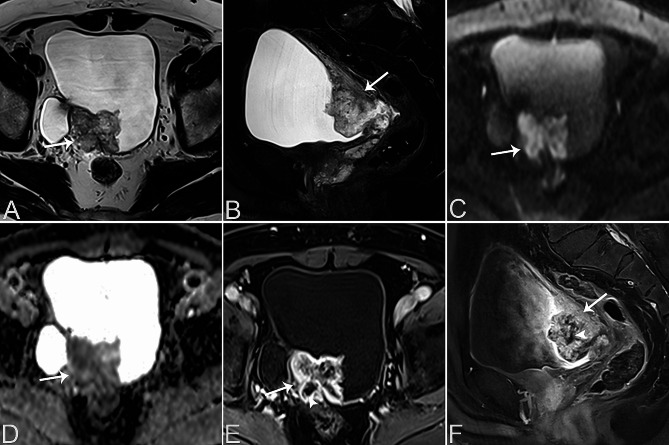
A 70-year-old male patient with bladder SUC in the right posterior wall of the bladder. Axial T2WI (A) and sagittal T2WI (B) show a lobulated mass in the right posterior wall of the bladder, demonstrating hyperintensity on T2WI (arrows). Axial DWI (C) shows the mass with high signal intensity (arrow). Axial ADC map (D) shows diffusion restriction of the bladder tumour (arrow). Contrast-enhanced axial (E) and sagittal (F) T1W images of the bladder tumor (arrows) displays heterogeneous and marked enhancement, with the presence of non-enhancing necrotic areas (arrowheads)
